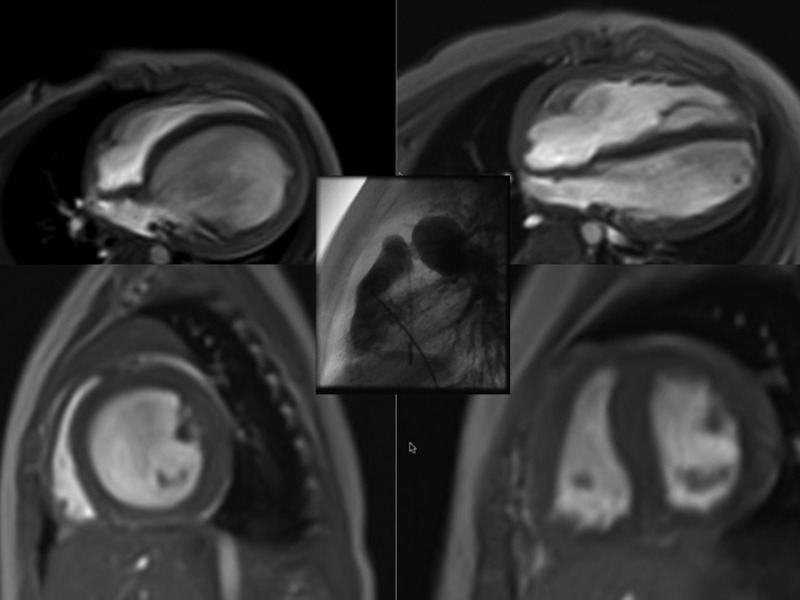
(see, online supplementary video 2a,b) Effects of a reversible pulmonary artery banding (rPAB) on the left ventricle in dilated cardiomyopathy (DCM). Depicted are the MRI of left-sided DCM in four chambers and sagittal views before and in the follow-up after a surgically performed PAB (picture in the middle); the progression of left-ventricular dilatation with its pathological ventriculo-ventricular interaction caused by the morphological change of the left ventricle from ellipsoid to a spherical left ventricle (LV)-form (like pear to apple) lead to a consecutively compressed right ventricular to a banana-shaped chamber associated with an early compromised diastolic inflow. The rationale to place a surgically rPAB is to induce a leftward mechanical shift of the interventricular septum back to the LV with a normally shaped left ventricle potentially resulting in an improved LV function.
